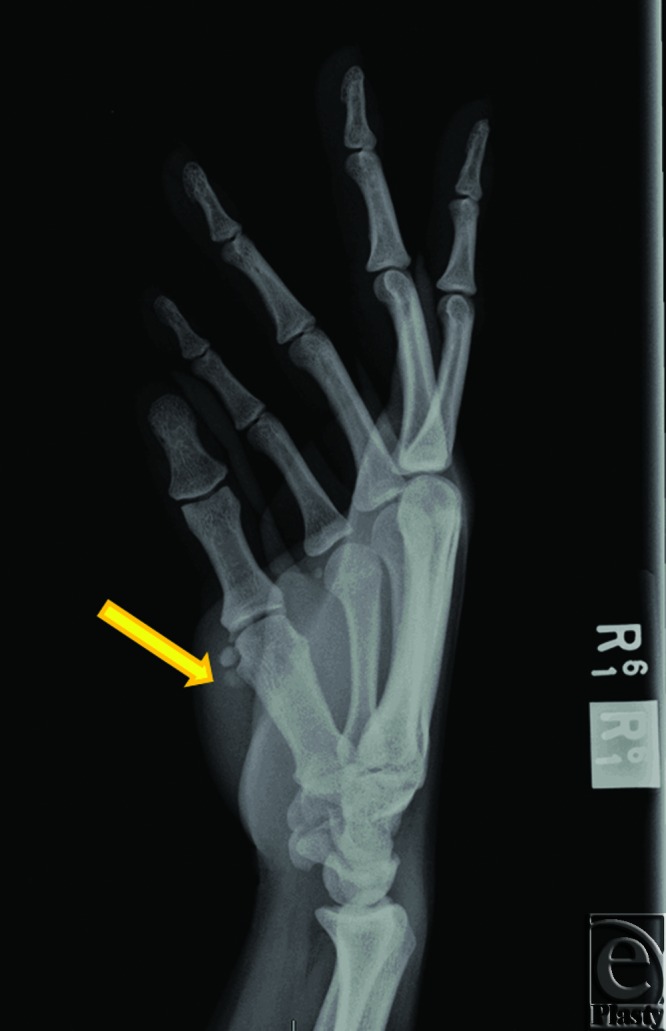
X-ray. Two 4-mm, radio-opaque masses (arrow) can be visualized alongside the volar and radial aspect of the head of the right first metacarpal bone. No acute fracture is noted.
